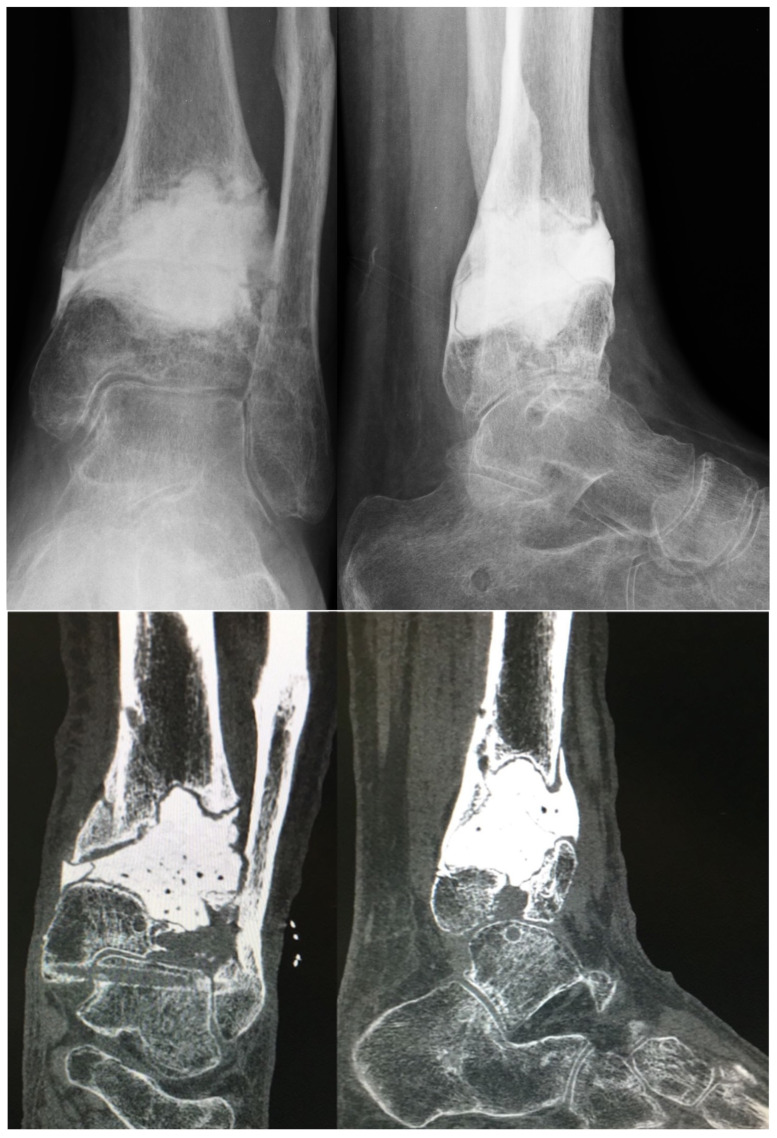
X-rays (above) and CT scan (below) of the previous case after wide surgical toilette, bone re-section, and positioning of an antibiotic-impregnated cement spacer.
